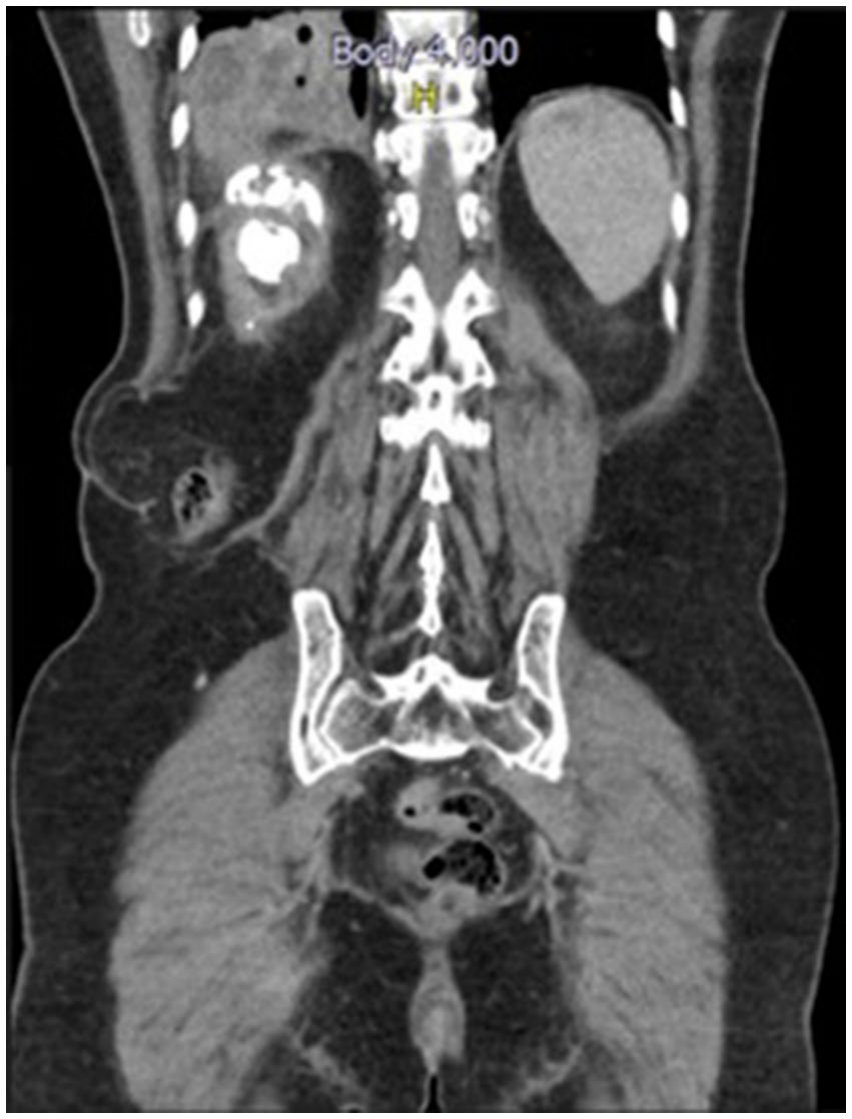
CT scan of the abdomen and pelvis with soft tissue contrast window sagittal view shows a coralliform calculus occupying all the right renal collectors with active fistula between the upper collector and the right basal lung parenchyma.
